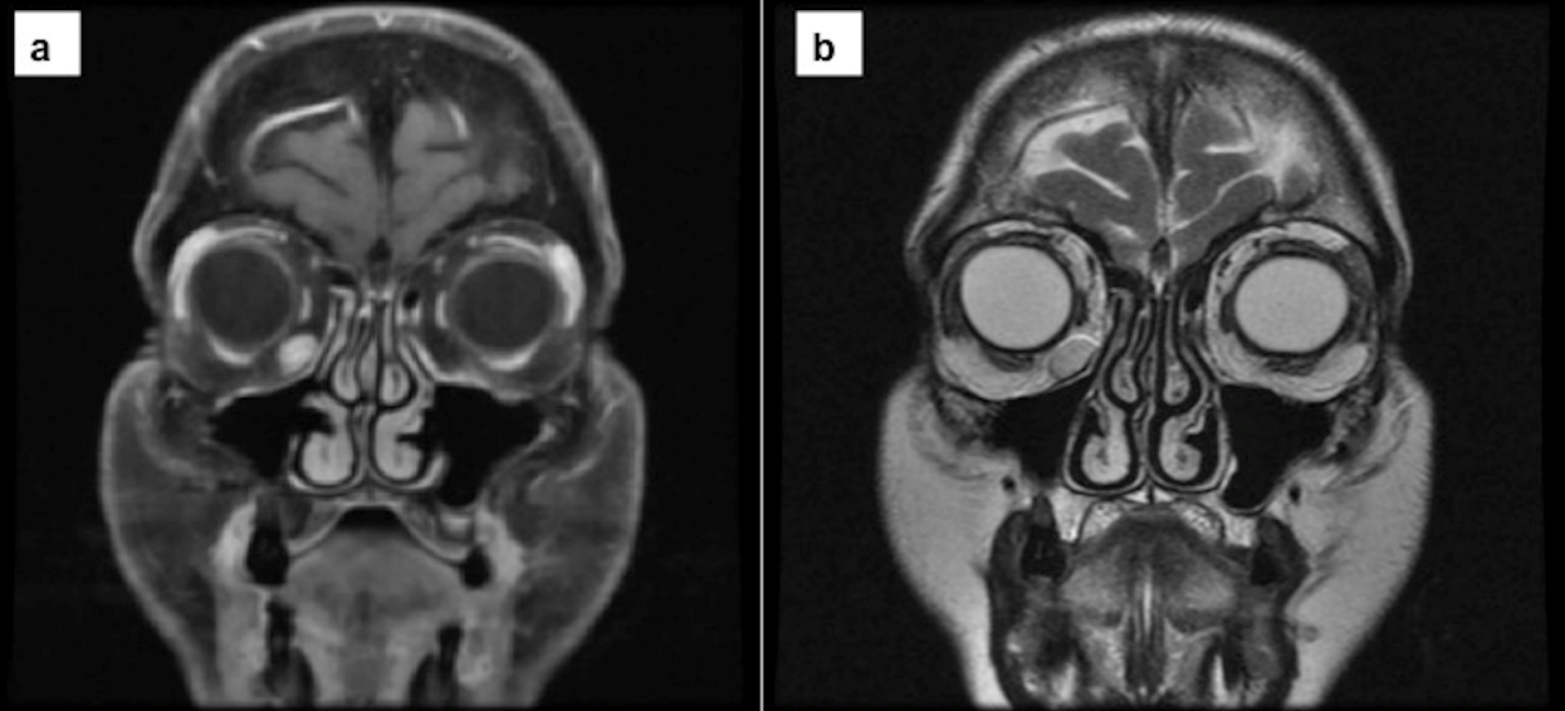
Magnetic resonance imaging of the coronal section. a) MRI – T1 gadolinium-enhanced showed a well-defined lesion in the inferior orbital rim that was enhanced with contrast. b) MRI – T2 showed a hyperintense lesion. The capsule is shown as well as low-intensity septation.
